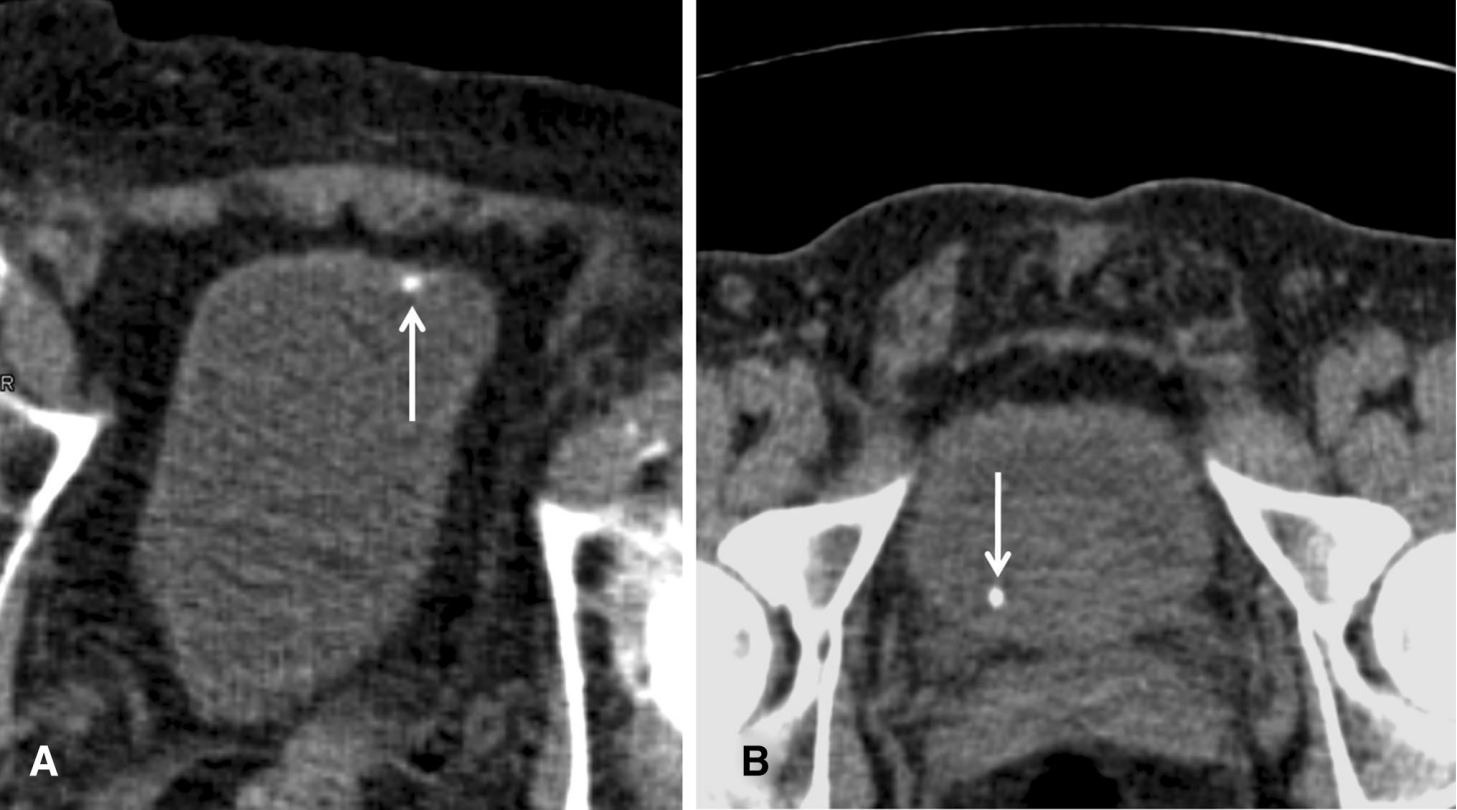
A, B Prone axial unenhanced CT images in two different patients with acute renal colic (images were flipped 180° and can be read in the usual way). Stone located anteriorly in the bladder in keeping with a ureteral stone that had already passed into the bladder (A, *arrow*). Intramural stone impacted at the ureterovesical junction not moving anteriorly on the prone scan (B, *arrow*)
